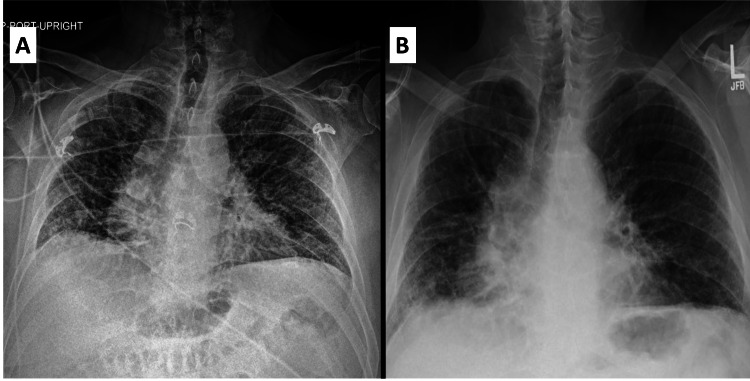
CXR showing evidence of interstitial lung disease and resolution. A, CXR on admission; B, CXR after 4 weeks of steroids CXR, chest X-ray
